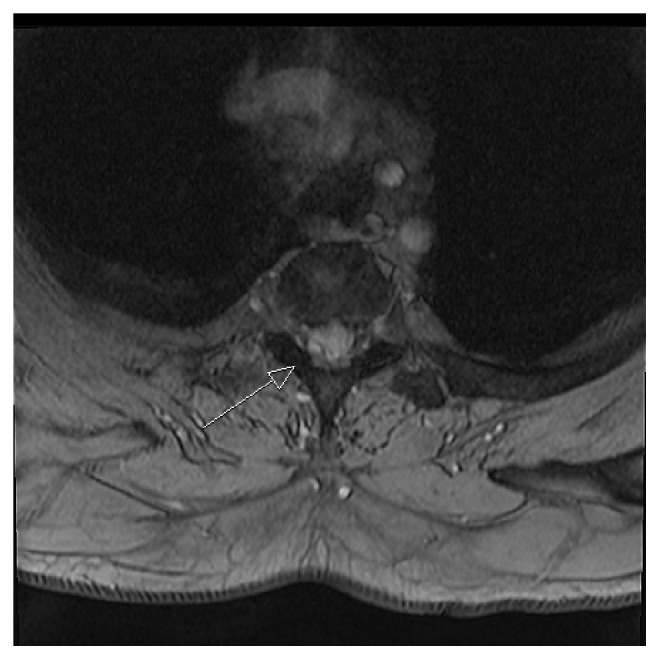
MRI of the upper spinal column T2-weighted sequence, transverse plane. White arrow indicating tumor location with compression of spinal cord.
